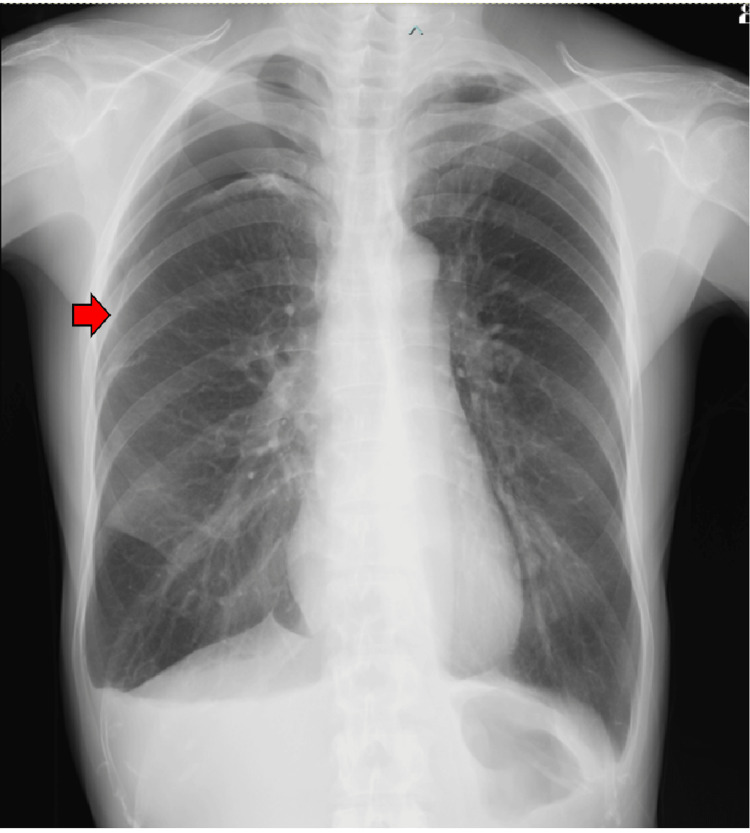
Chest radiography on postoperative day 16 Enlargement of the space in the right lung apex is visible, with progression of the right lung collapse (red arrow)
